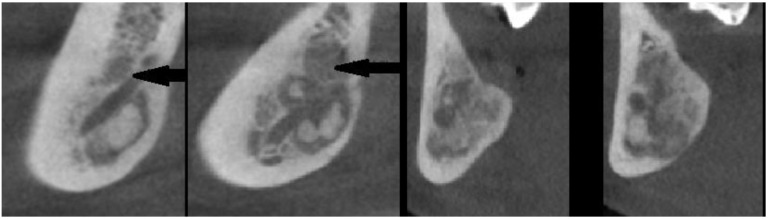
Sagittal CBCT projections of COD surrounding the inferior alveolar nerve without any visible compression or changes in the bony canal shape and position. Black arrow points on the mandibular canal and the lesion located within the bone canal.
